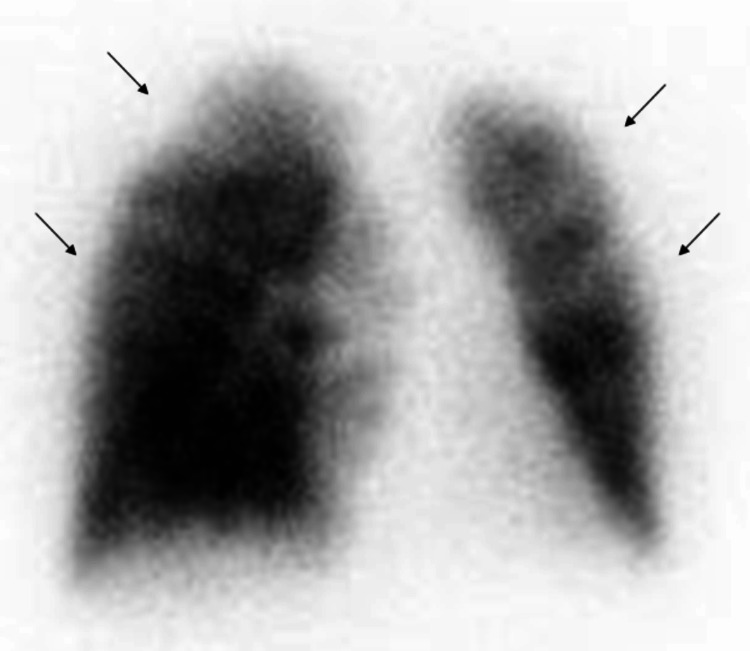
Lung perfusion scan. Bilateral multiple wedge-shaped perfusion defects in lung perfusion scintigraphy (arrows).
